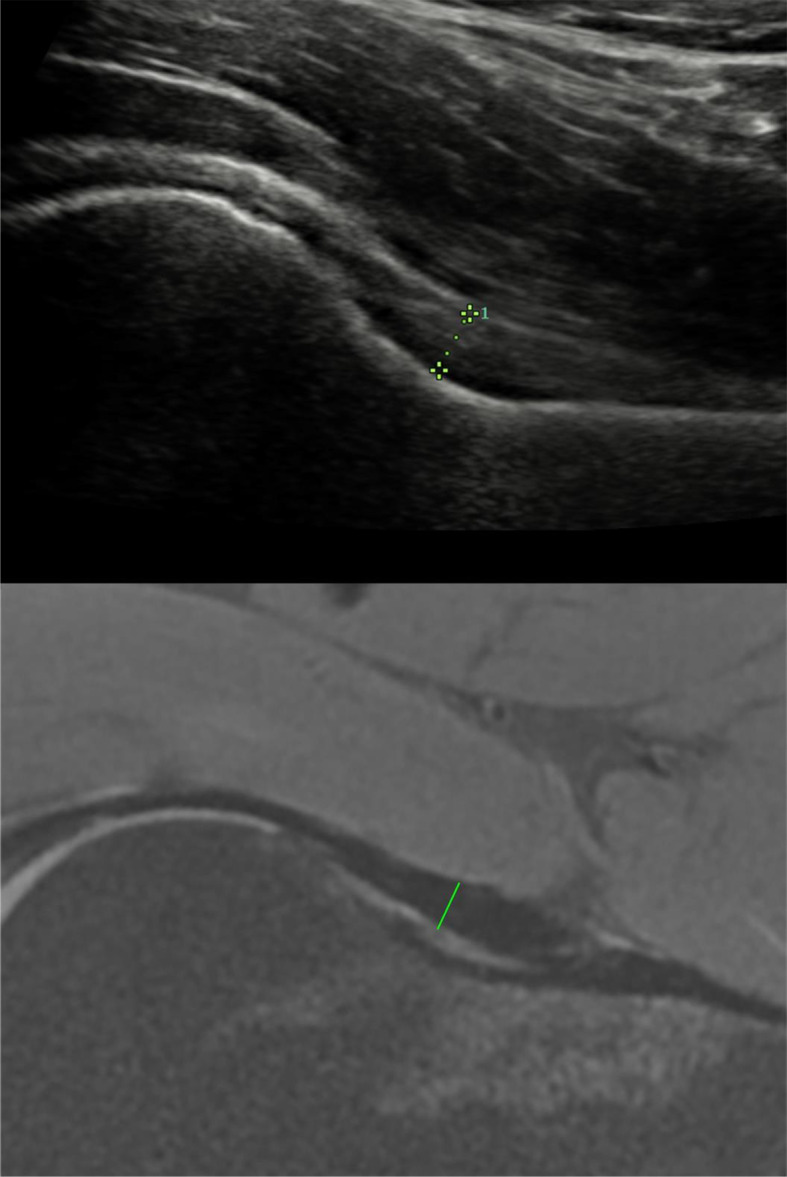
Left hip capsule thickness measurement of same patient on MRI and ultrasound
